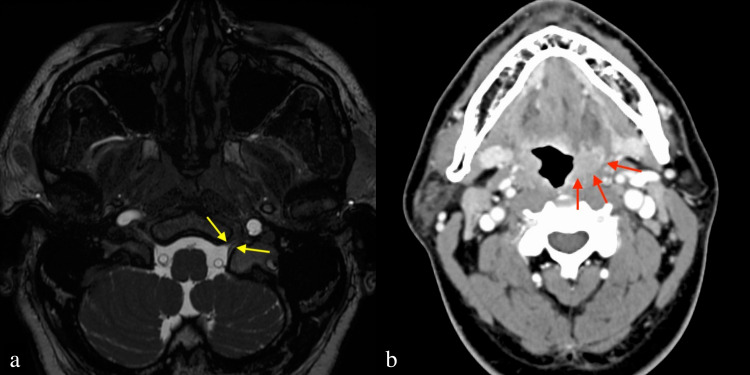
MRI and contrast-enhanced computed tomography (CECT) images at the initial visit (a) MRI image showing no notable lesion found nearby the left hypoglossal canal (yellow arrows). (b) CECT image showing swelling of the left lingual tonsils and palatine tonsils (red arrows), but no neoplastic lesions were found. CISS: constructive interference in steady-state
